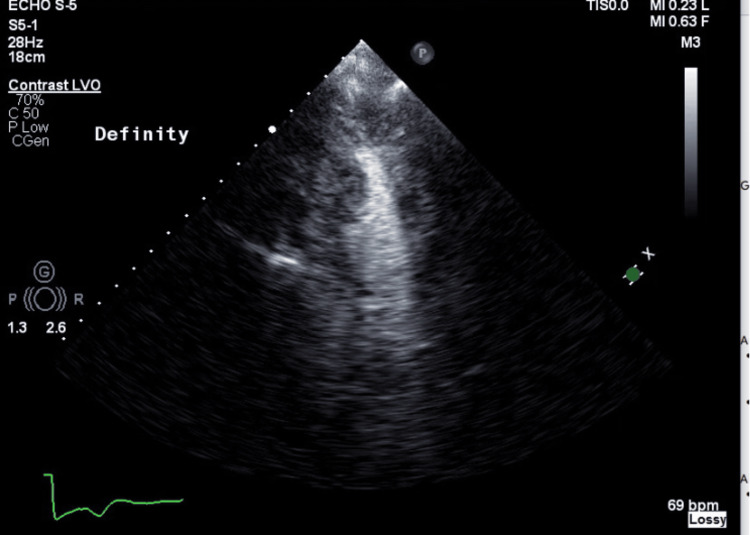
Echocardiogram showing apical four chamber view. In view is the left ventricle with mildly increased left ventricular wall thickness at the base with more prominent hypertrophy in the apical segments.
